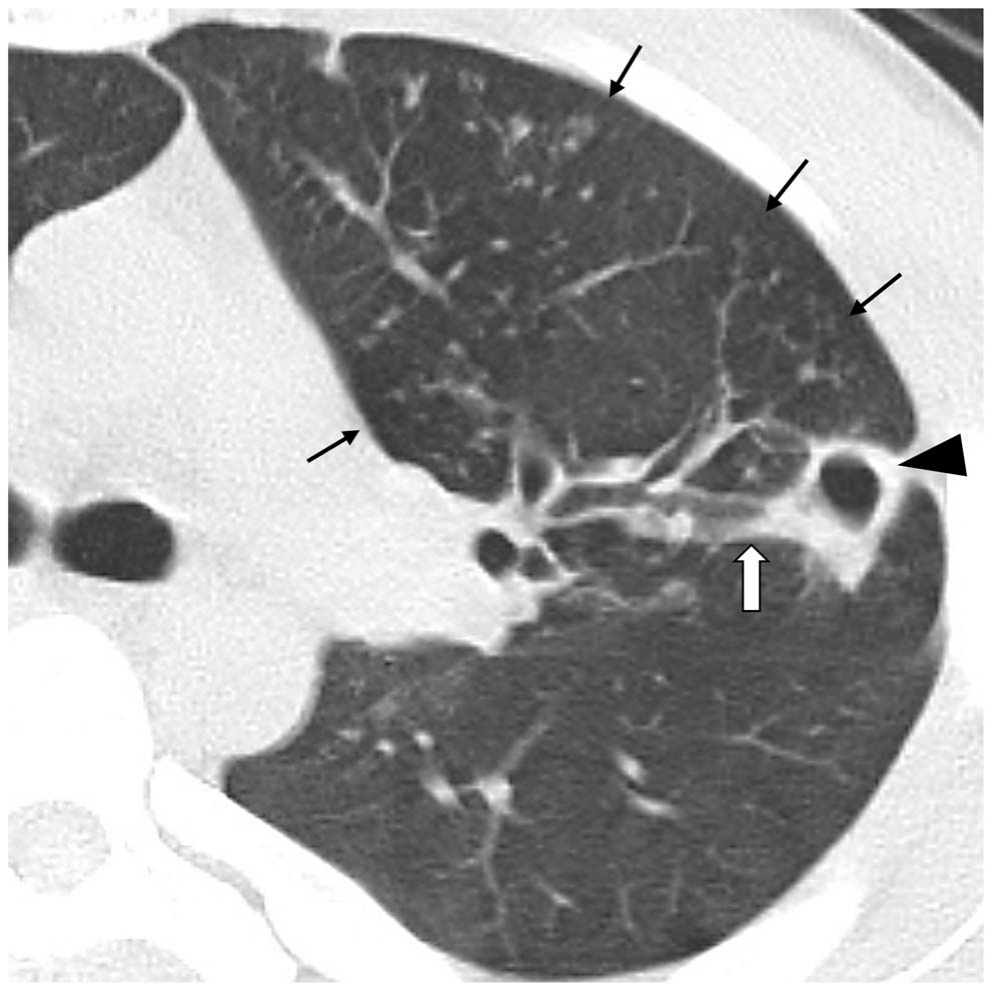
CT findings of active TB. A chest CT image of the left upper lobe of the lung in a 19-year-old subject demonstrated clustered non-calcified nodules associated with dilated peripheral airways (thin arrows) and a cavitation (arrow head) with dilated and thickened subsegmental bronchus (white arrow).
